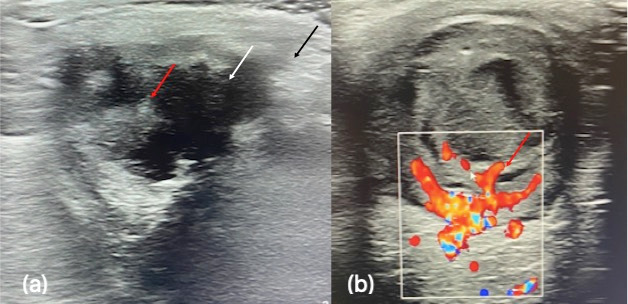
Orbital ultrasound. (a) Showed the alteration of the morphology of the eyeball with thickening and alteration of the echogenicity of the covers. It also showed occupation of the vitreous chamber by heterogeneous hyperechogenic material (*red arrow*) and solution of continuity of the temporal ocular wall (*white arrow*) with outflow of the contents and adjacent collection (*black arrow*). (b) Doppler study showed destructuring of the posterior wall with vascularized intraocular membranes in relation to probable retinal and choroidal detachment (*red arrow*).
